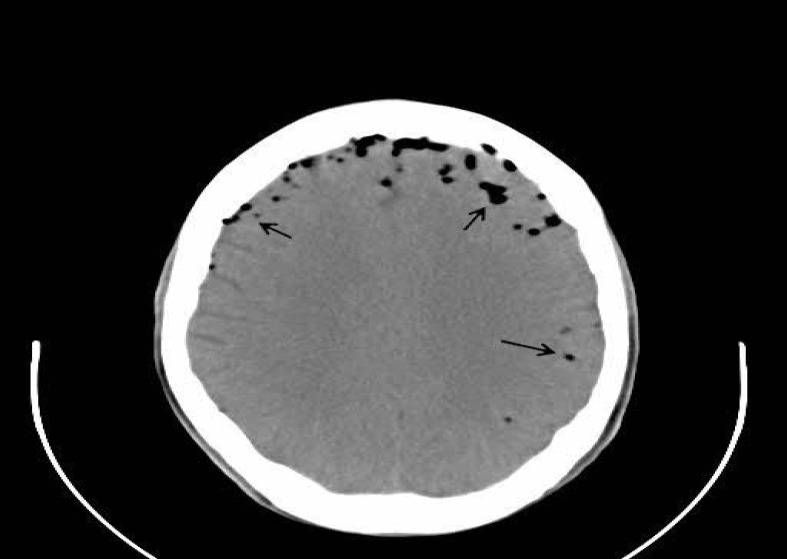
There were large amounts of air bubbles in the brain CT scan
